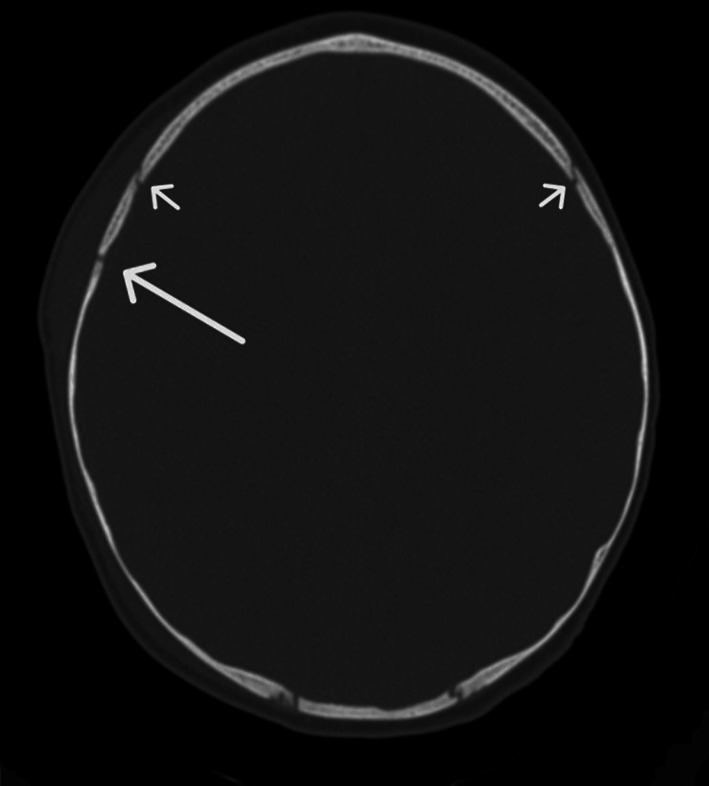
Axial CT scan without contrast of the brain reveals a compound skull fracture (indicated by the arrow) and a hematoma in the right parietal area
